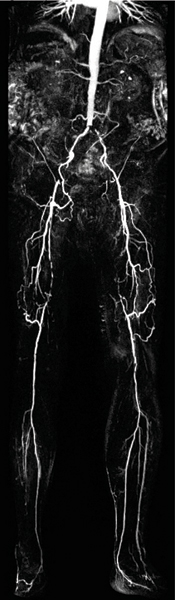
CT-MRA dataset with excellent image quality without any venous enhancement or artefacts. Clear depiction of several atherosclerotic changes like occlusion of the superficial femoral artery on both sides.
